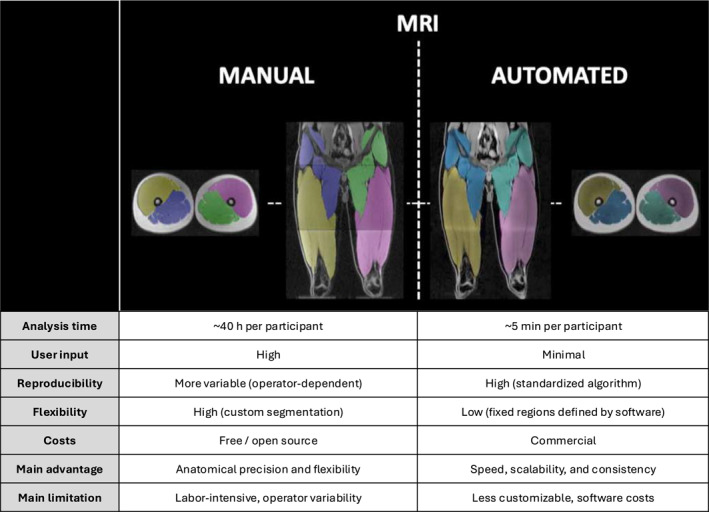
Transverse/axial and coronal images of the manual (left) and automated (right) MRI segmentation techniques. For manual segmentation, MRI images were segmented and colored manually using ITK‐SNAP software. For automated segmentation, MRI images were processed using AMRA Researcher (AMRA Medical AB, Linköping, Sweden), which applies a nonrigid multi‐atlas segmentation technique to automatically analyze thigh muscle volume, with manual quality control. Both methods included segmentation of the anterior and posterior muscle compartments. A summary table highlighting the key differences between manual and automated analysis is included directly below for reference.
